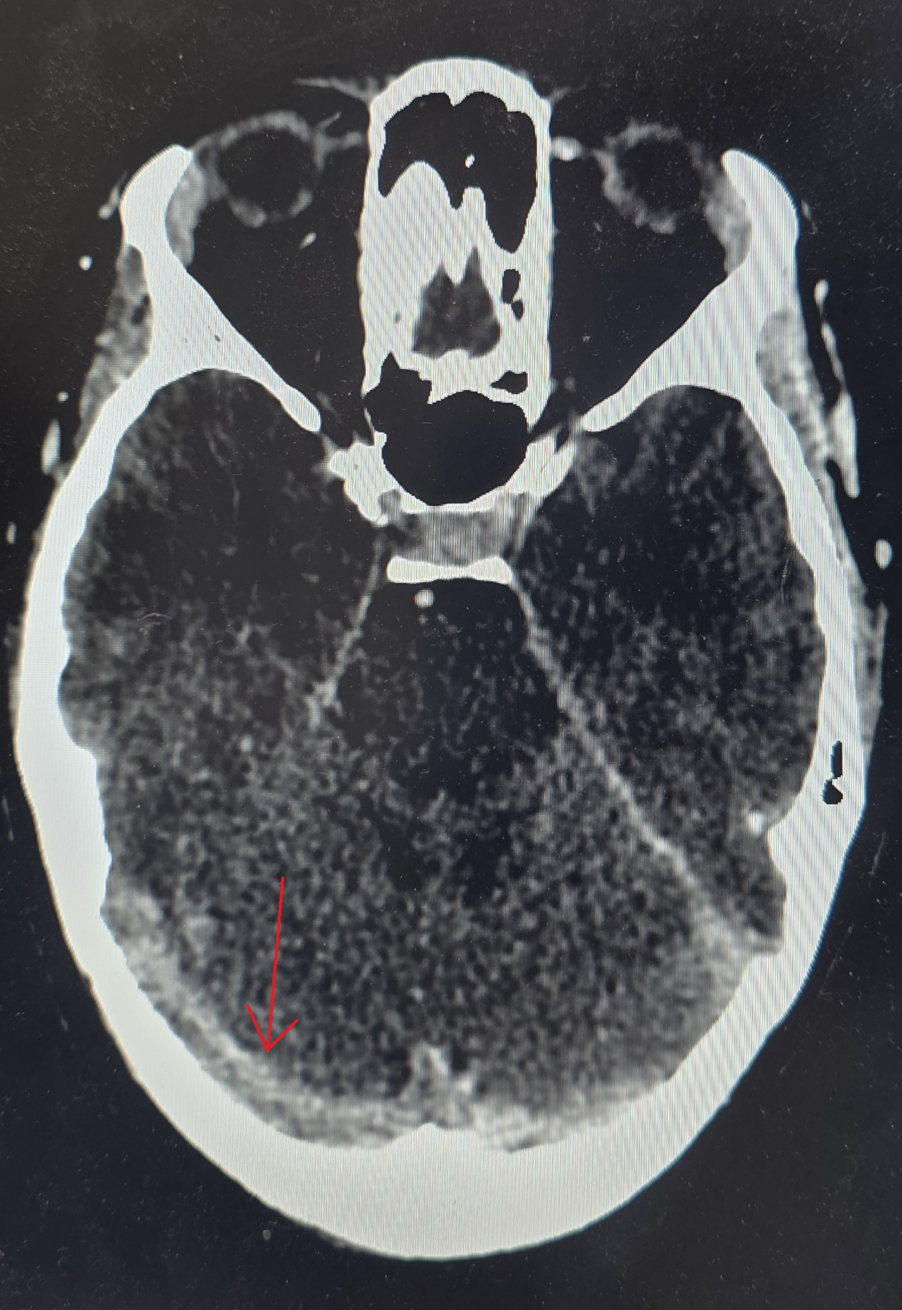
Cerebral CT angiography showing cerebral venous thrombosis of the right transverse sinus (arrow) CT: computed tomography
